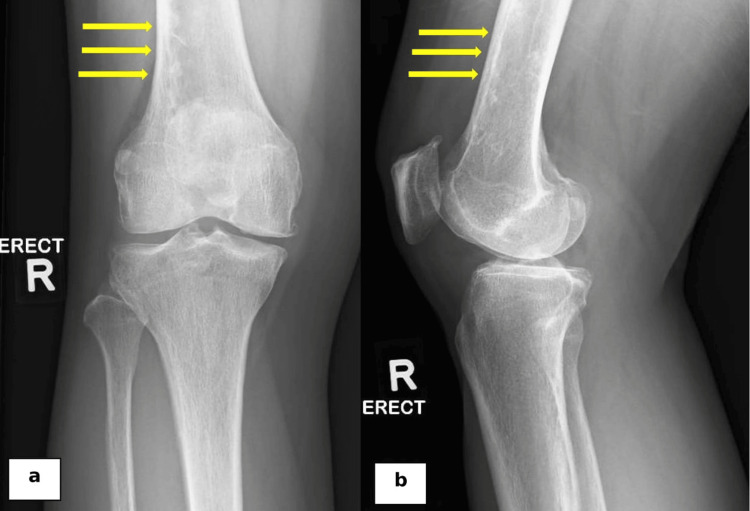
Plain radiographs of the knee in anteroposterior (a) and lateral (b) views revealed tri-compartmental osteoarthritic changes along with a suspicious elongated lucent medullary lesion in the femoral shaft (yellow arrows).
